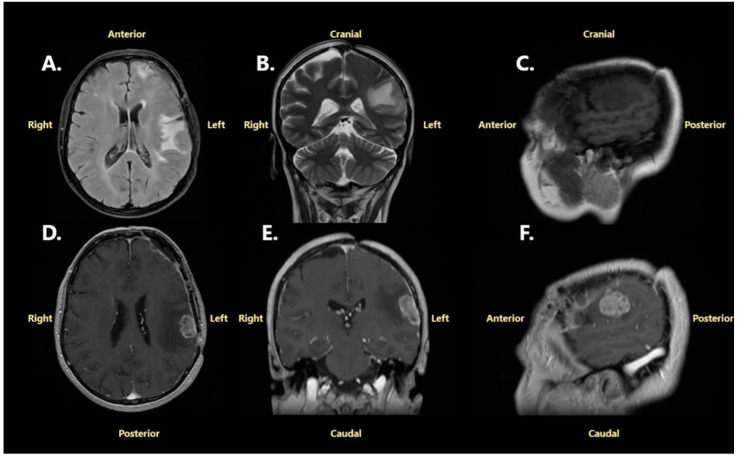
Brain MRI performed in July 2024: (A)—fluid-attenuated inversion recovery with fat saturation (FLAIR FS) T2-weighted sequence, axial view; (B)—T2 FSE sequence, coronal projection; (C)—T1-weighted image, sagittal projection; (D)—T1-weighted MPR sequence with contrast enhancement, axial projection; (E)—T1-weighted MPR sequence with contrast enhancement, coronal projection; (F)—T1-weighted MPR sequence with contrast enhancement, sagittal projection.
